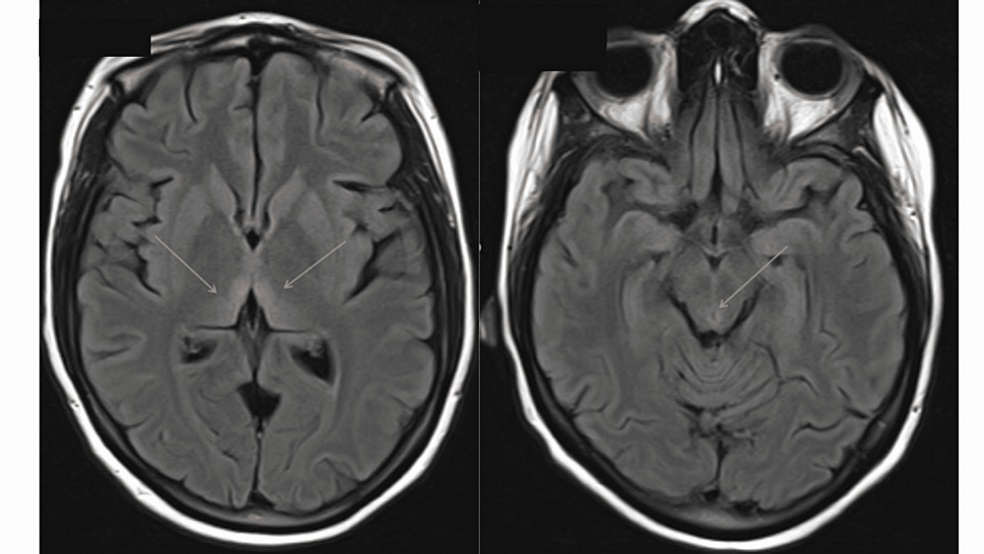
MRI of the brain with and without contrast. MRI brain showing T2 flair hyperintensities in dorsomedial thalamus (left) and periaqueductal region (right), consistent with the presumed diagnosis of Wernicke’s encephalopathy. MRI: magnetic resonance imaging
